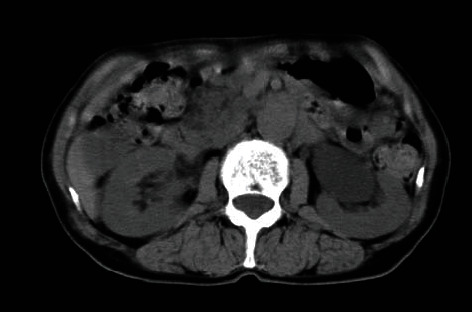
CT shows hydronephrosis of the left lower-pole.
